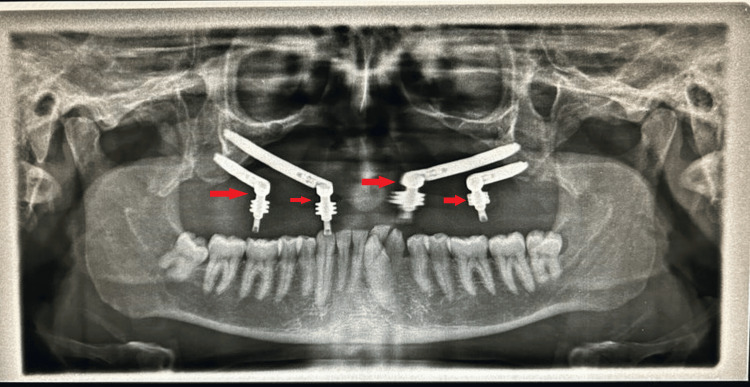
Orthopantomogram showing zygomatic implants Arrows are indicative of four zygomatic implants with abutments placed
